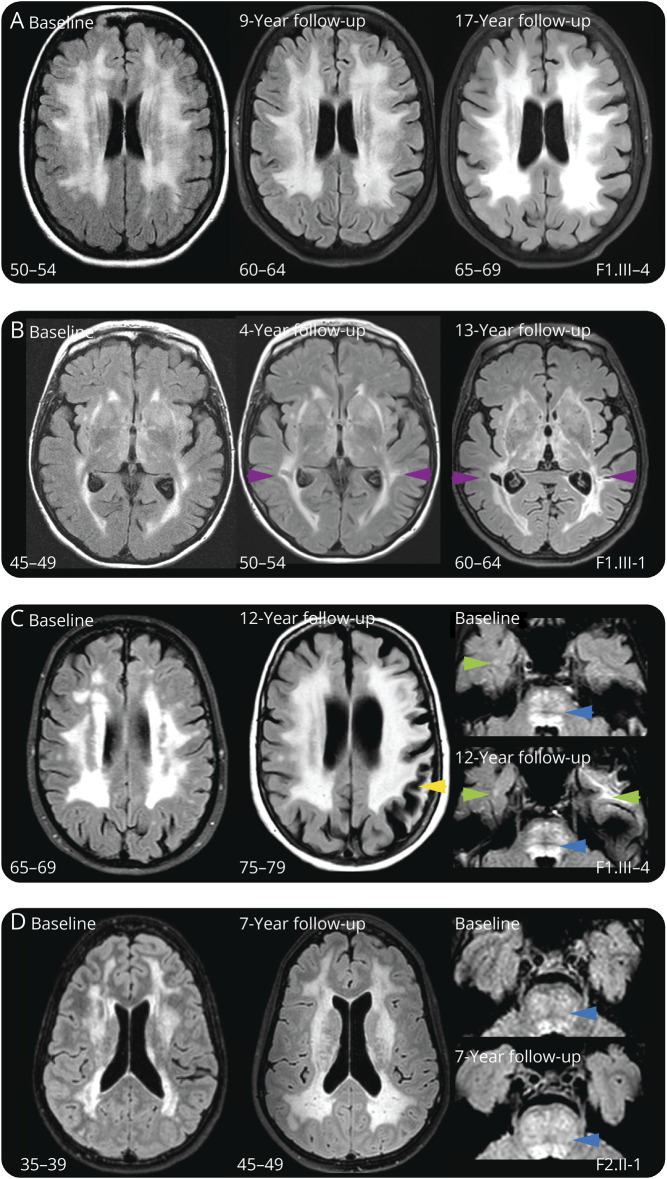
Disease Progression in Patients With CARASAL To ensure patient anonymity, only age ranges were given. (A–D) 4 examples of long-term progression of MRI abnormalities in patients with CARASAL. (A) T2-FLAIR: a 50–54-year-old female patient with CARASAL with striking WMHs at baseline, which remained relatively stable over a follow-up period of 17 years. No lacunes developed in or near these extensive WMHs; a small (silent) lacune in the thalamus became visible after 9 years of follow-up (not shown). (B) T2-FLAIR: a 45–49-year-old female patient with CARASAL with confluent WMHs at baseline, which further increased during a 13-year follow-up. Silent lacunes developed near periventricular WMHs (purple arrowheads). (C) T2-FLAIR: a 65–69-year-old female patient with CARASAL with highly confluent WMHss at baseline. This patient developed an infarct in the perfusion area of the left medial cerebral artery at 12 years of follow-up (yellow arrowhead). Although calcifications of the bifurcation of the internal carotid arteries (ICAs) were present, no significant stenosis (<25% stenosis) of the ICA was found, with no signs of atrial fibrillation. This patient had no lacunes on MRI, nor were there CMBs present. Highly confluent pontine white matter hyperintensities remained stable over a 12-year follow-up period (blue arrowheads). The patient had punctate WMHs in the right ATL (left green arrowhead), likewise, without evident change during the 12 years of follow-up. The WMH in the left ATL (right green arrowhead) developed after the aforementioned cortical stroke. (D) T2-FLAIR: a 35–39-year-old female patient with CARASAL with highly confluent WMHs at baseline with an increase during a follow-up period of 7 years. This patient did not develop infarcts or CMBs. Extensive hyperintensities in the pons (blue arrows) were already present from a young age (<40) and increased further with age. CADASIL = cerebral autosomal dominant arteriopathy with subcortical infarcts and leukoencephalopathy; CARASAL = cathepsin A–related arteriopathy with strokes and leukoencephalopathy; CMBs = cerebral microbleeds; FU = follow-up; WMHs = white matter hyperintensities.
